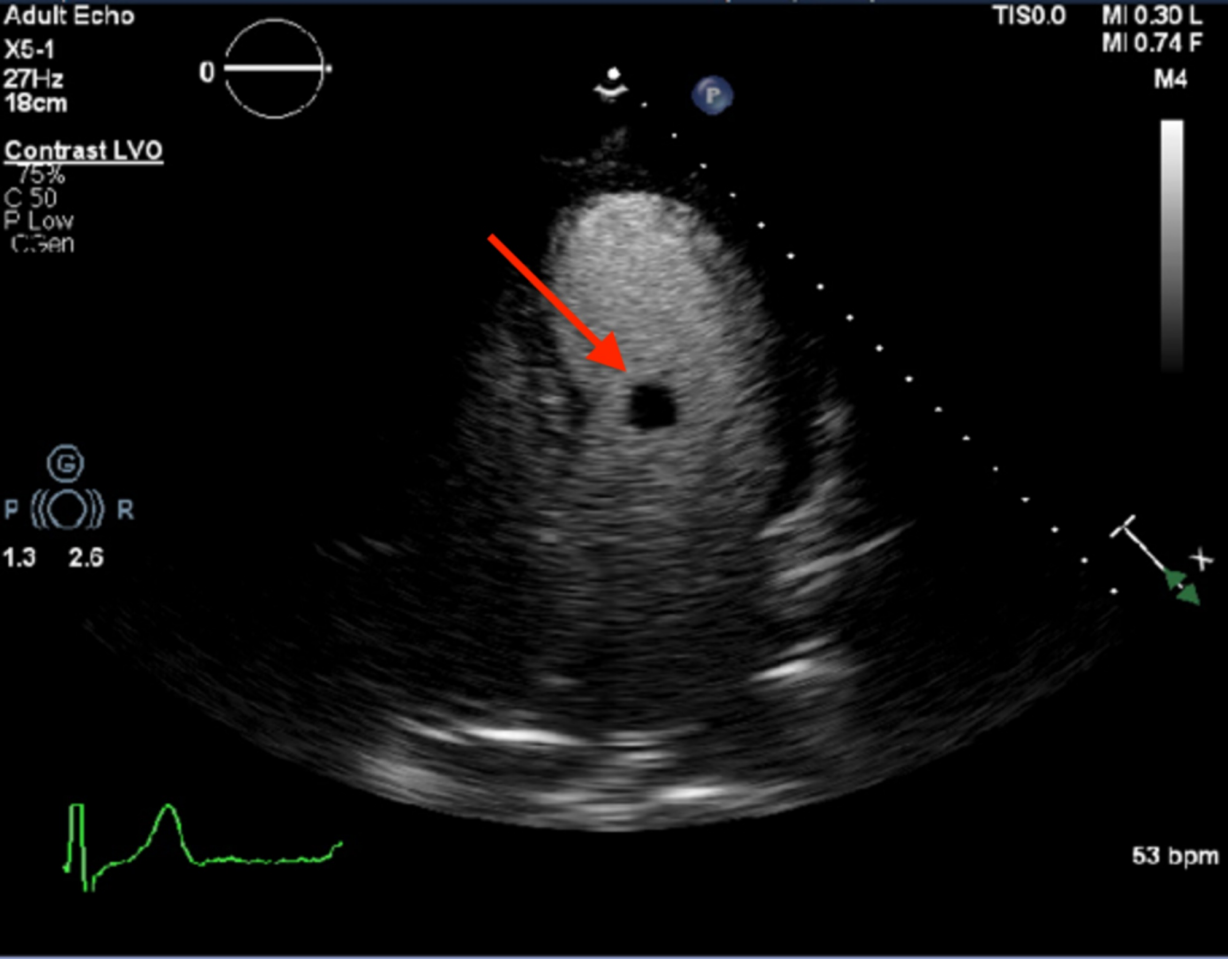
Apical four chamber view of two-dimensional transthoracic echocardiogram at the time of presentation An Echocardiogram showing 11 x 9.5 mm circular echolucent spherical mobile mass at the subvalvular mitral valve apparatus, on the left ventricular aspect (red arrow).
